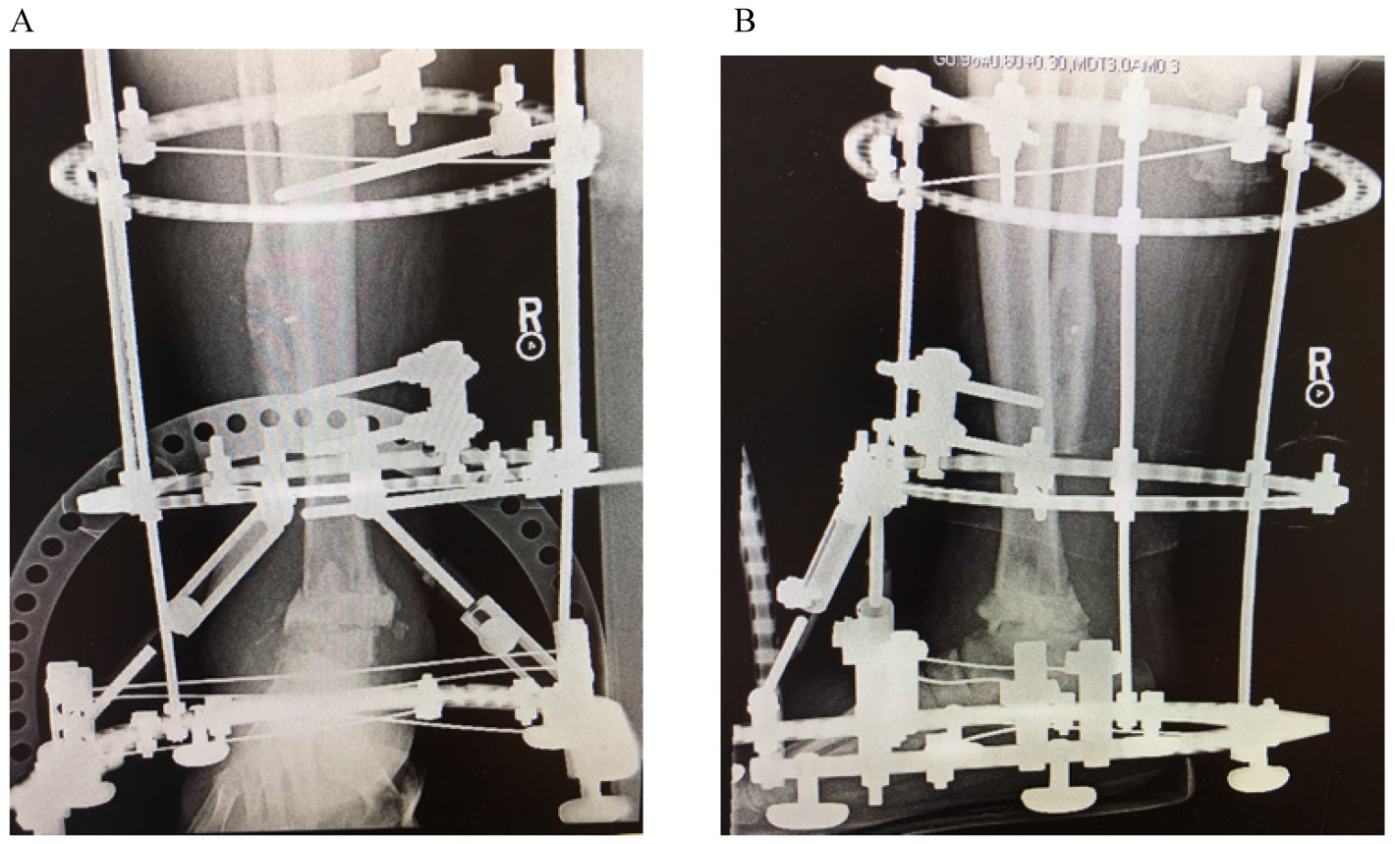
AP (A) and lateral (B) radiographs following removal of hardware, placement of tibiotalar antibiotic spacer and ringed external fixator
